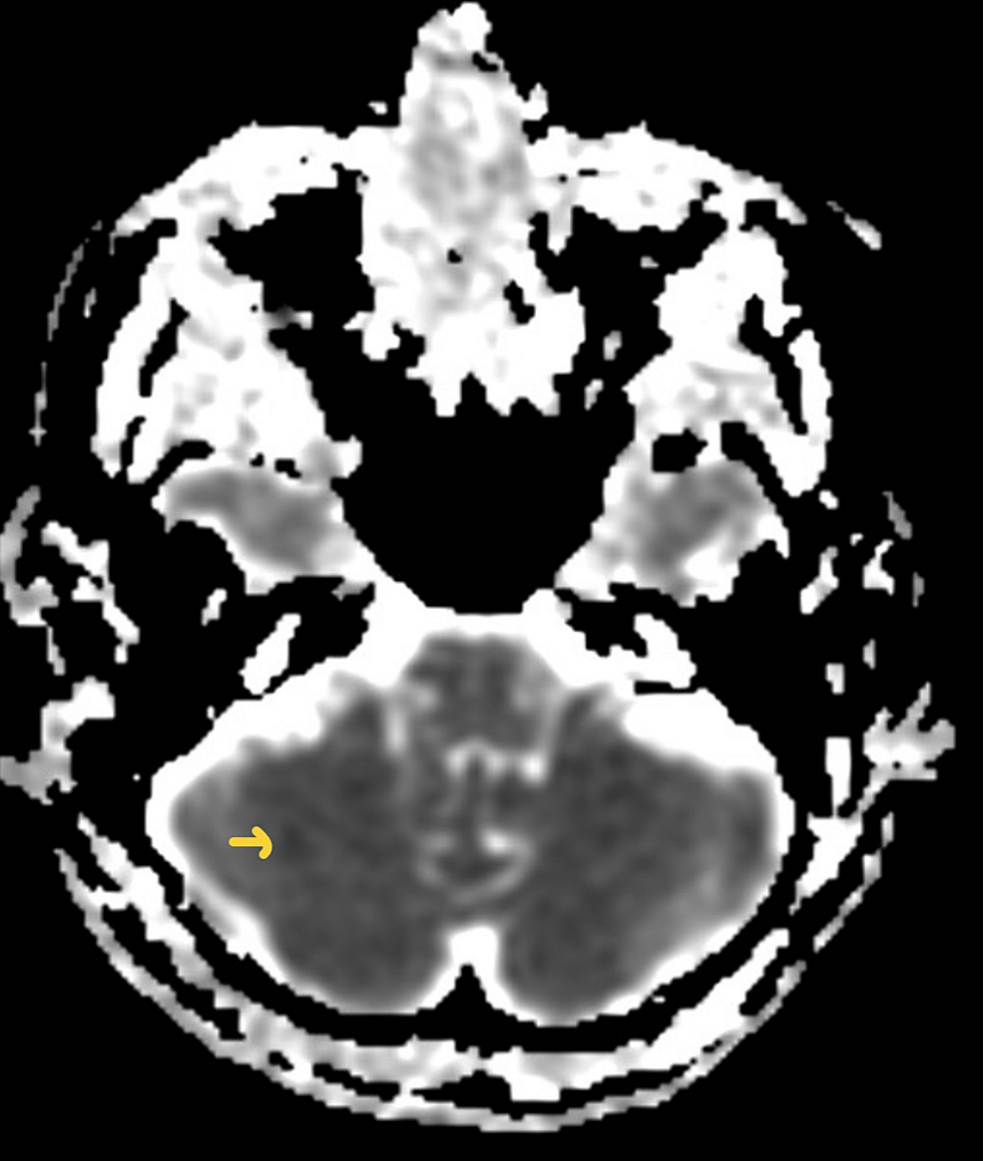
Magnetic resonance imaging with apparent coefficient mapping (MRI-ADC) showing cerebellar infarct (arrow)
